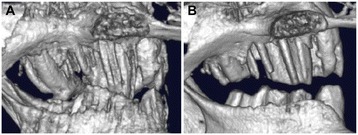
CT images of elongated premolars and molars, taken before and after treatment. (A) A case of excessively long premolars and molars. (B) Image taken after treatment.
